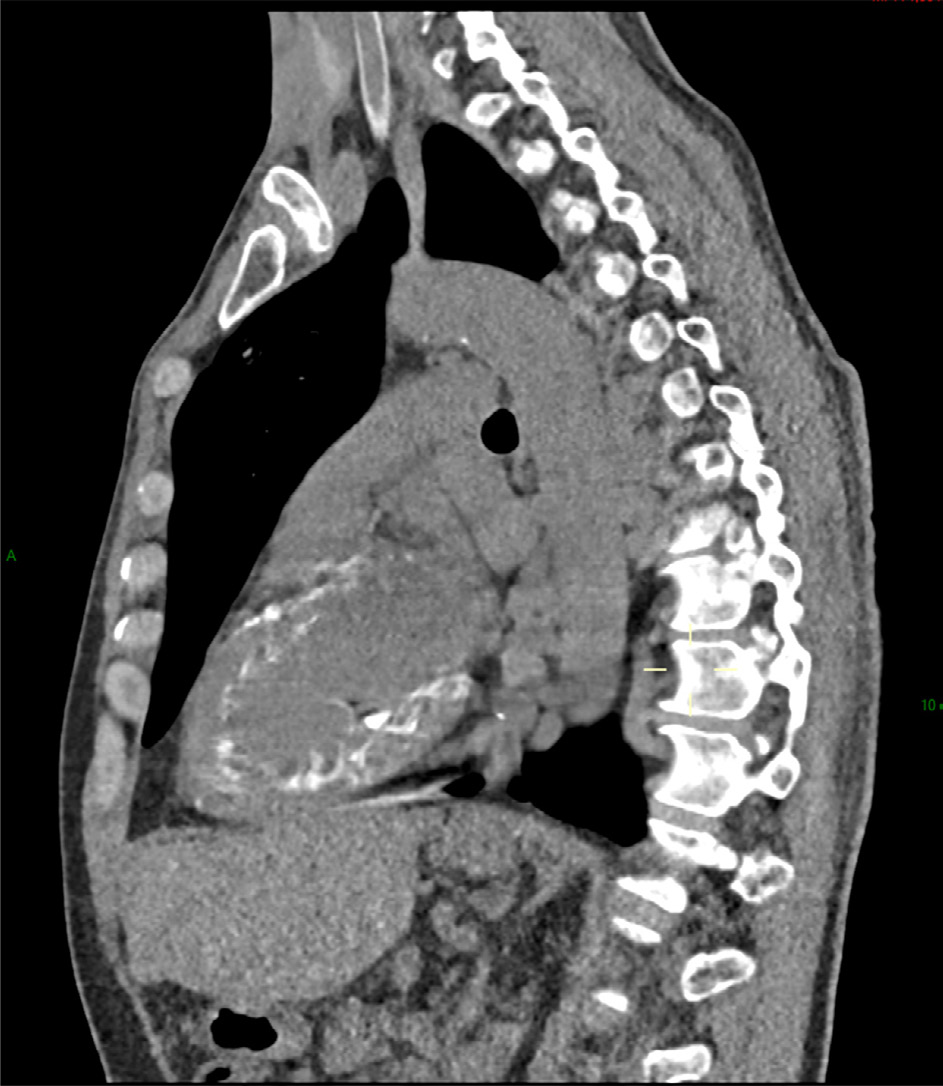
Sagittal chest CT: parietal left cardiac ventricle calcifications (porcelain heart).
